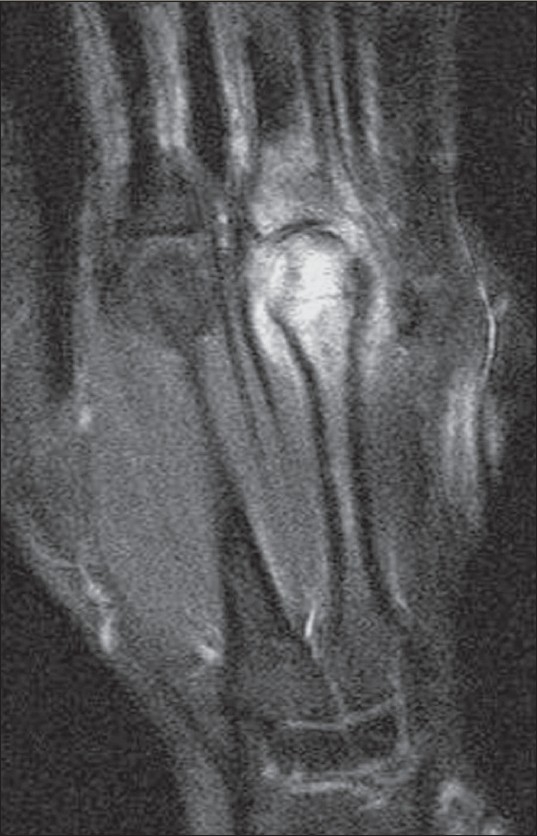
Tuberculous arthritis of the metacarpophalangeal joint. Coronal, fat-suppressed T2W MRI shows hyperintense bone marrow edema on both sides of the third metacarpophalangeal joint with joint fluid effusion. The margin of the subchondral cortical bone of the head of the metacarpal bone is indistinct
